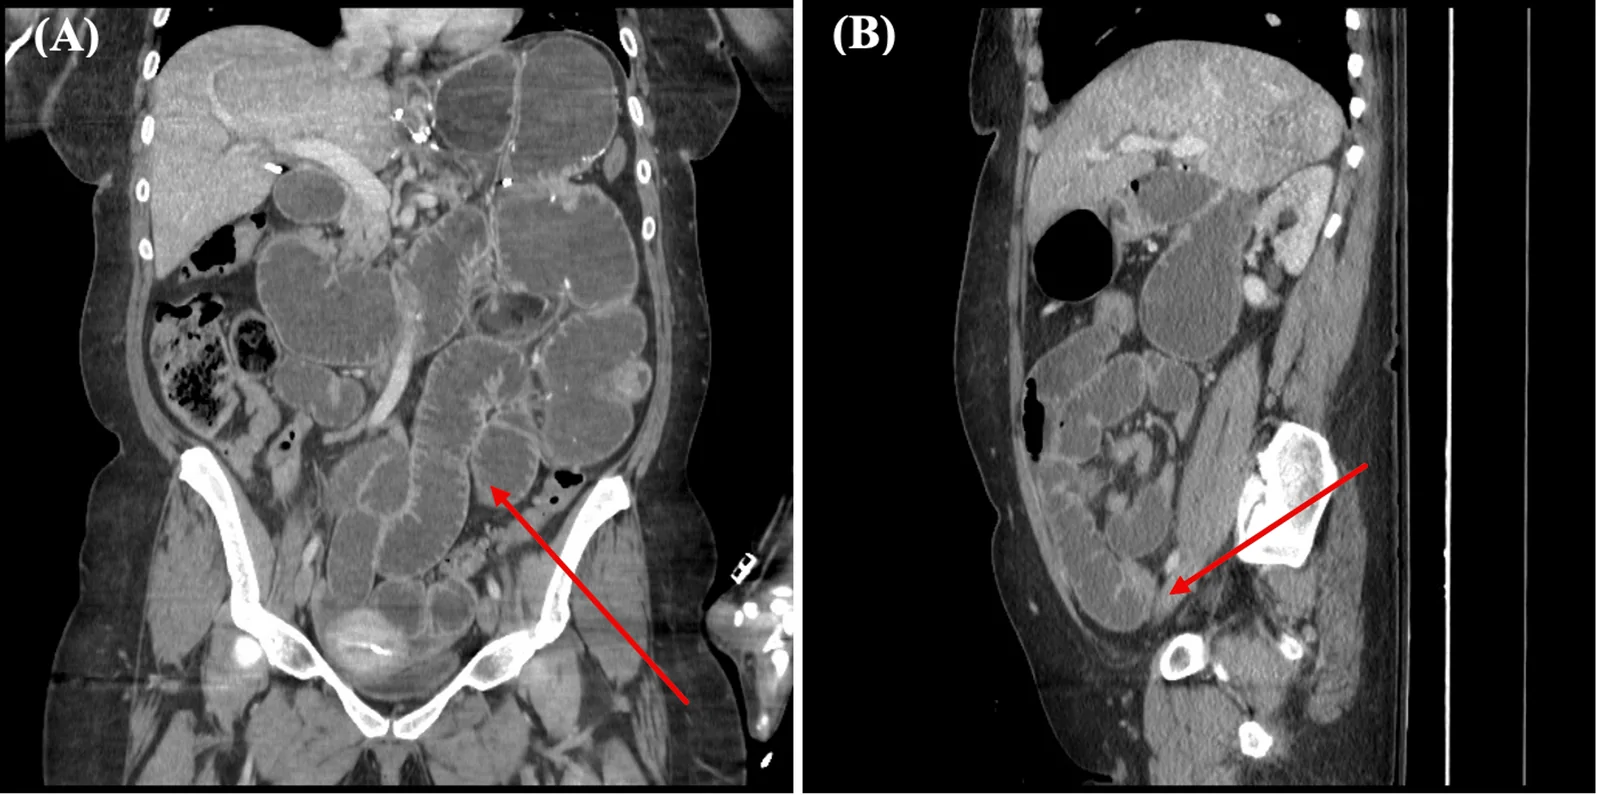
Contrast-enhanced CT of the abdomen and pelvis demonstrating findings consistent with small bowel obstruction. (A) Coronal CT image demonstrating multiple dilated, fluid-filled small bowel loops (arrow), consistent with small bowel obstruction. (B) Sagittal CT image demonstrating dilated, fluid-filled small bowel loops (arrow). A discrete transition point and the underlying etiology of the obstruction were not clearly identified on preoperative imaging.
